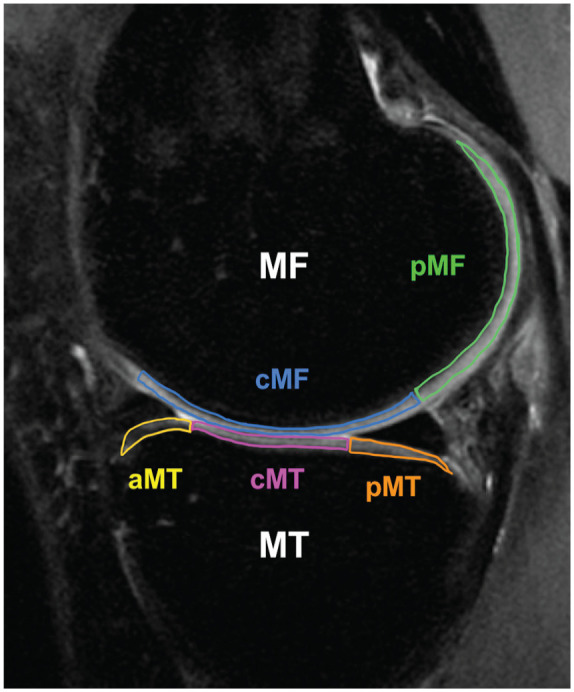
Segmentation of the sagittal magnetic resonance imaging scan of the medial compartment. The medial femur (MF) and medial tibia (MT) are divided into subcompartments with respect to the meniscus. aMT, anterior MT; cMF, central medial femur; cMT, central MT; pMF, posterior medial femur; pMT, posterior MT.
